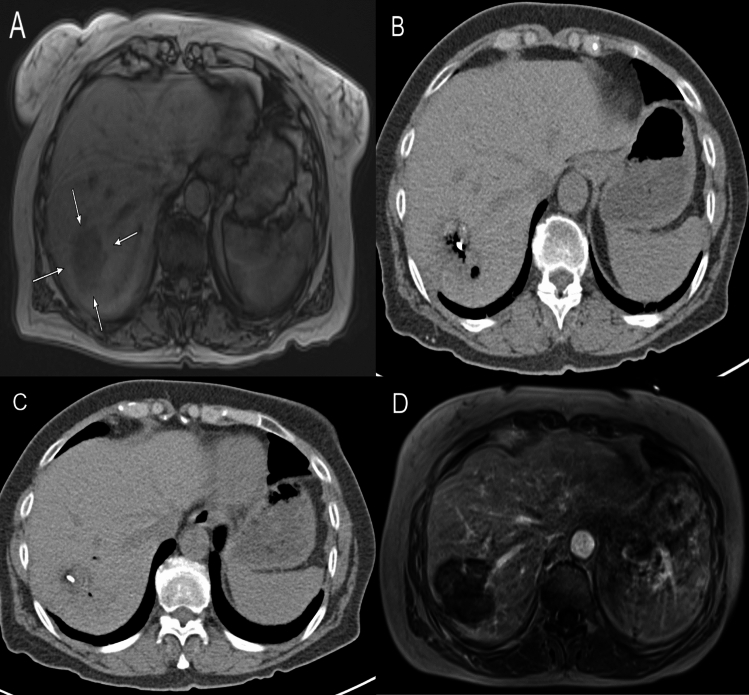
A patient with LMCRC treated by TACE followed by MWA. A MRI shows a LMCRC (white arrows) in the right liver lobe; B,C During MWA ablation, where some air bubbles can be seen; D MRI scan 24 h after MWA shows the ablation zone
